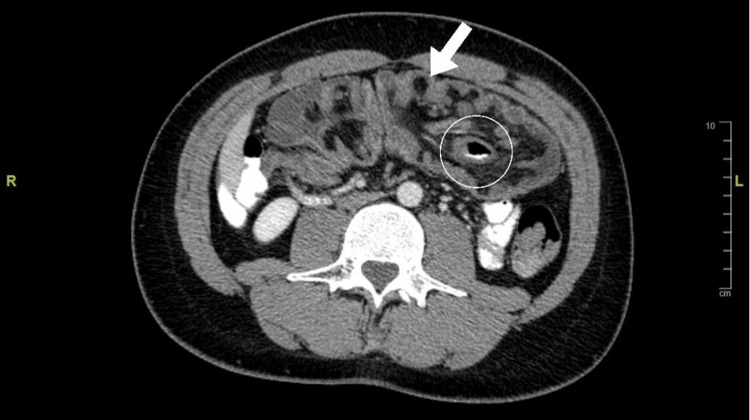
CT scan of the abdomen and pelvis without contrast showing long segment intussusception marked by the arrow and lead point marked by the circle
